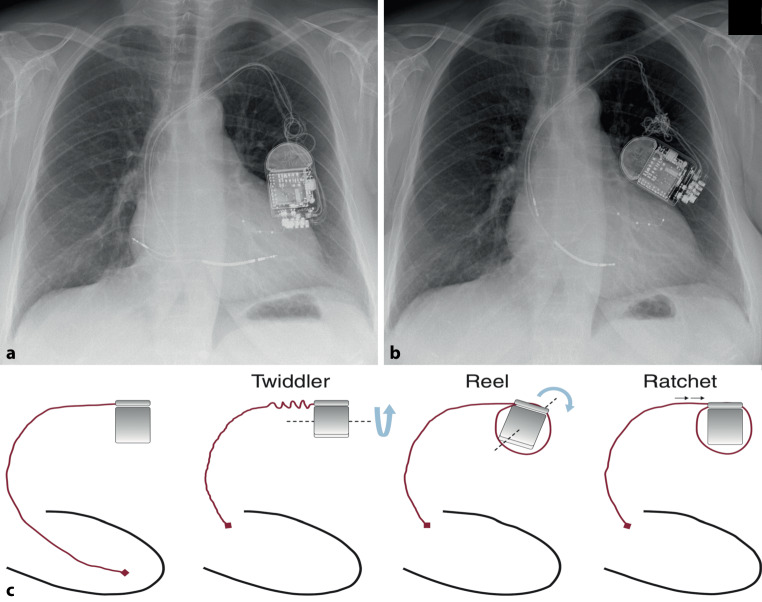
a Chest radiography taken four months earlier for an unrelated purpose, already showing a rotated generator with some lead twisting. However, it was not reviewed by a cardiologist at that time. b Chest radiography at presentation, revealing both Twiddler and Reel syndromes, with leftward rotation of the pulse generator, CRT‑D lead twisting, and dislodged atrial and right ventricular leads. The left ventricular lead remained properly positioned. The intense twisting and progression between (a) and (b) suggest compulsive generator manipulation by the patient. c Movements involving the generator or leads contributing to various macro-dislodgement syndromes. Reproduced with permission from Elsevier [1] ©. Not covered by this article’s CC BY 4.0 License. Reuse requires Elsevier’s permission
